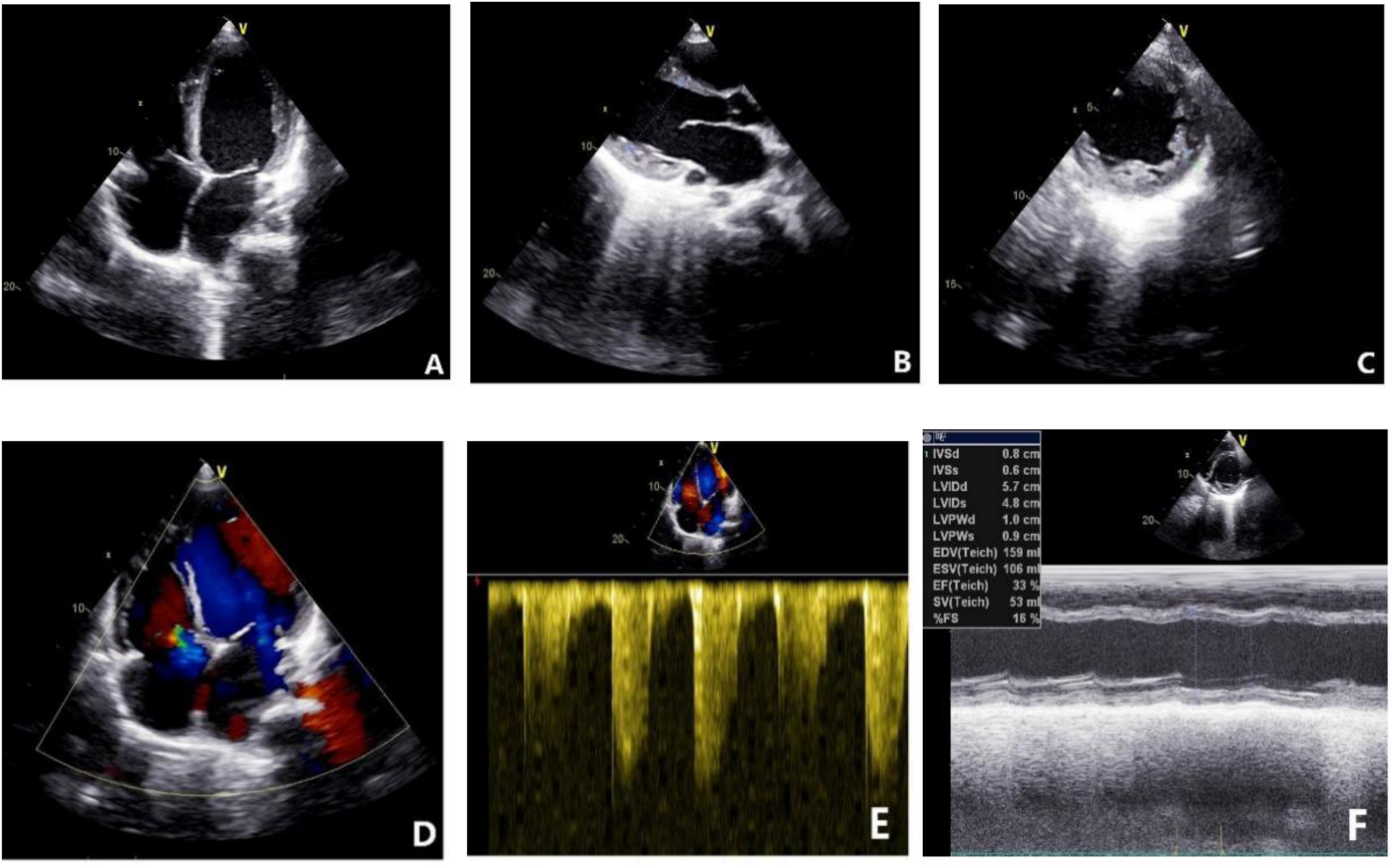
Echocardiogram results on October 14, 2021. (A) Four-chamber view showing global enlargement of the heart. (B) Long-axis view of the left ventricle indicating a left ventricular end-diastolic diameter of 51.60 mm. (C) Increased number of trabeculations in the mid to lower segments of the left ventricular lateral wall, with a trabecular height of 8.5 mm at the lateral wall. (D) Moderate regurgitation of both the mitral and tricuspid valves. (E) Indirect estimation of pulmonary artery systolic pressure of 40 mmHg. (F) Reduced cardiac function with an ejection fraction (EF) of 33%.
